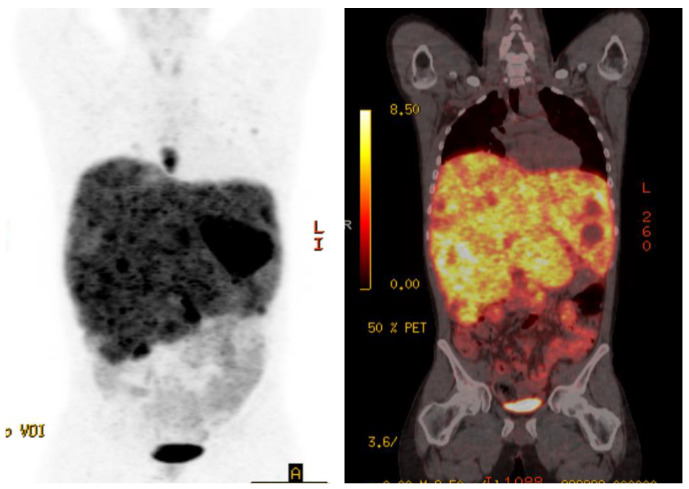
Case 1, Baseline Gallium-68 Dotatate PET-CT showed avid lesions in the lungs, posterior mediastinum, liver, retroperitoneal adenopathy, and diffuse liver and lung metastases.
